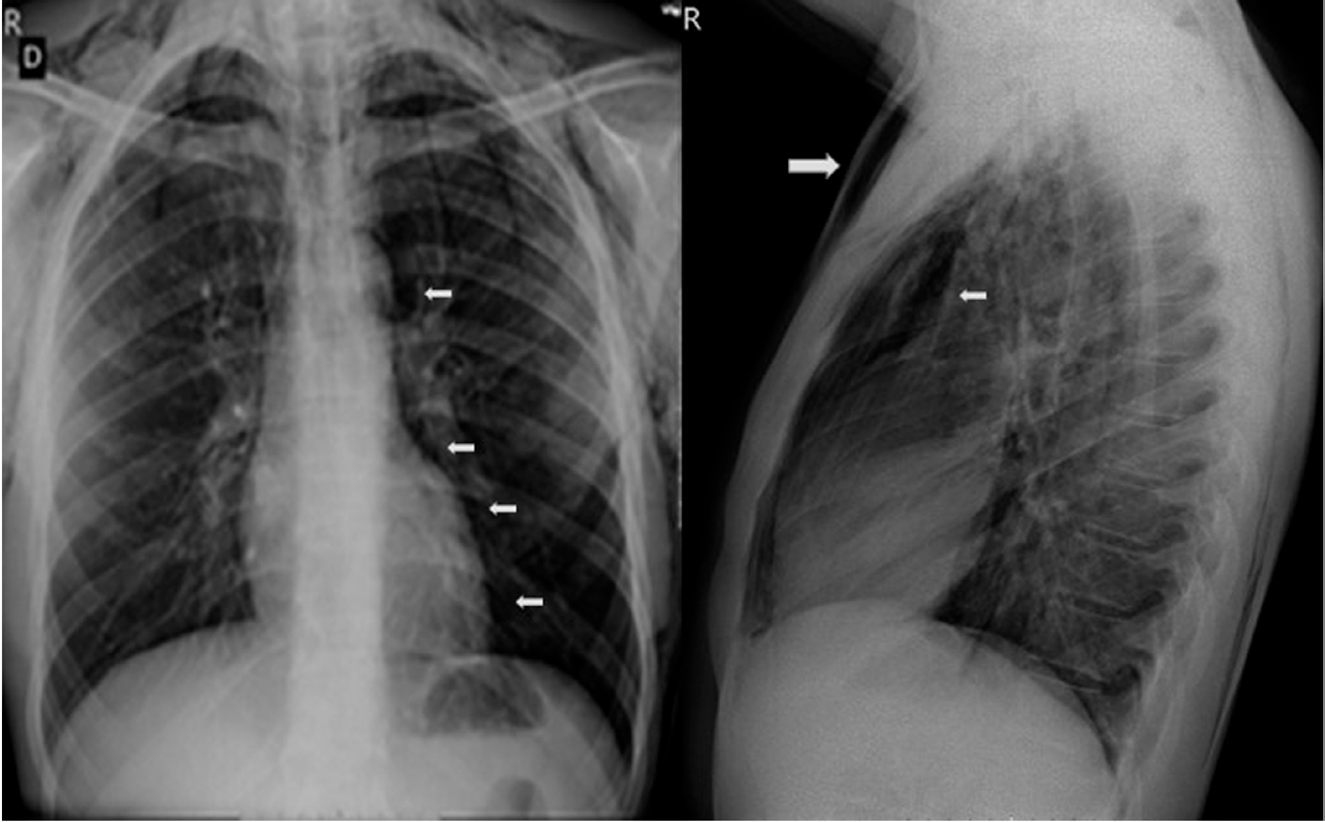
Chest X-ray at admission. A. Evidence of cervical and thoracic subcutaneous emphysema predominantly in the anterior region (large arrow). B. Pneumomediastinum extending into the deep cervical planes and pneumomediastinum (smaller arrows).
